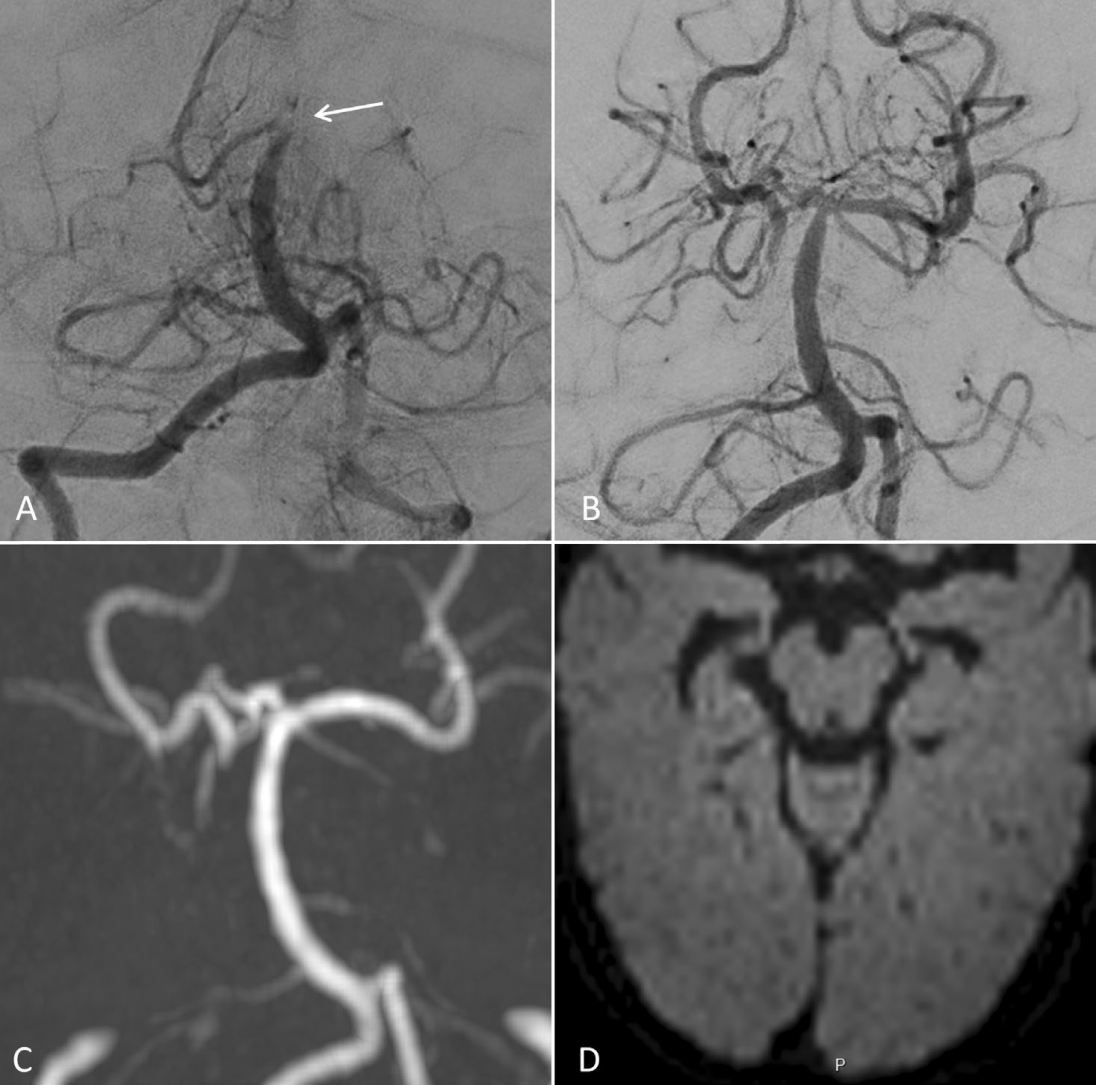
Patient 2, 5‐months‐old. Clot embolus at the top of the basilar artery (A: Arrow) treated by direct thromboaspiration through a Sofia 5F catheter with an eTICI score of 3 after 1 pass (B). MRA the day after (C) showed a patent basilar artery with no diffusion restriction on DWI (D). The pedNIHSS was 0 when the patient woke up after the intervention.
